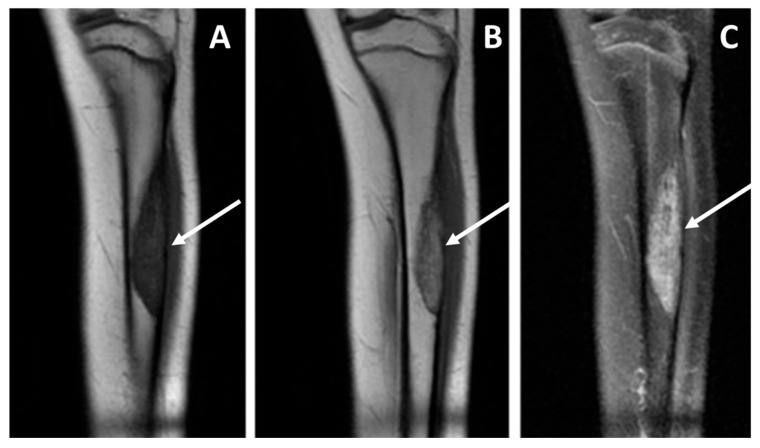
Baseline MRI study, coronal views, T1 weighted sequence (panel (A)), T1 weighted after contrast media injection (panel (B)), and fluid-sensitive sequence T2 weighted fat-sat (panel (C)), performed in a pediatric patient affected by adamantinoma. The study showed an intra-osseous lesion of the tibial diaphysis without a detectable extra-skeletal component (and consequently no vascular contact), and without any peritumoral edema. The patient did not develop metastatic disease; currently there is no evidence of disease more than 10 years after diagnosis.
